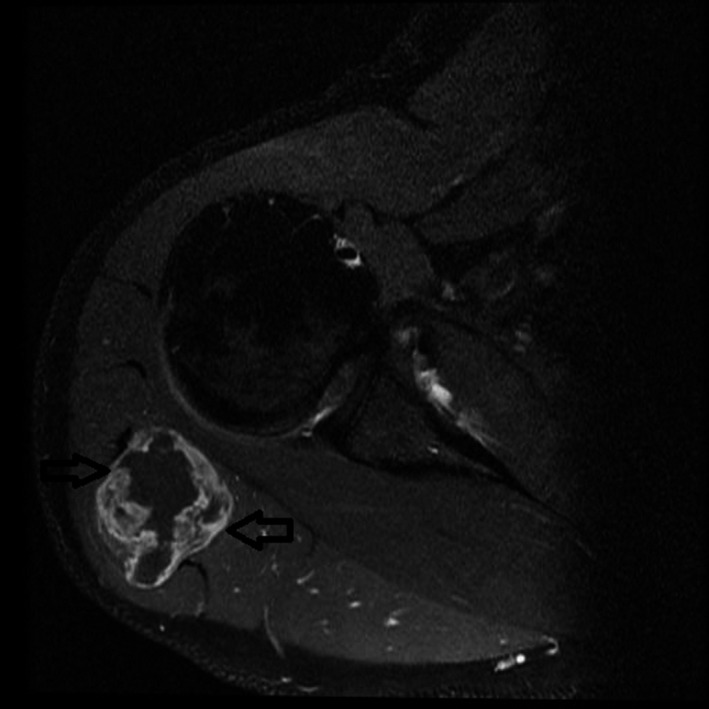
The MRI T2 sequence coronal plane shows polycystic tumor with solid aspects
